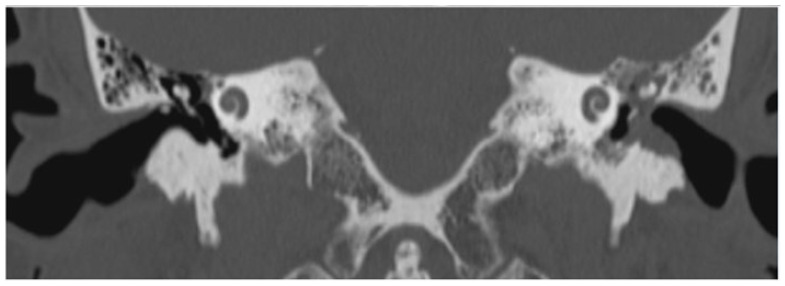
Patient 1, CT scan: evidence of soft tissue density located in the hypotympanic region of the middle ear cavity and in the floor of the left EAC with related bone erosion of the anteroinferior wall of the EAC.
